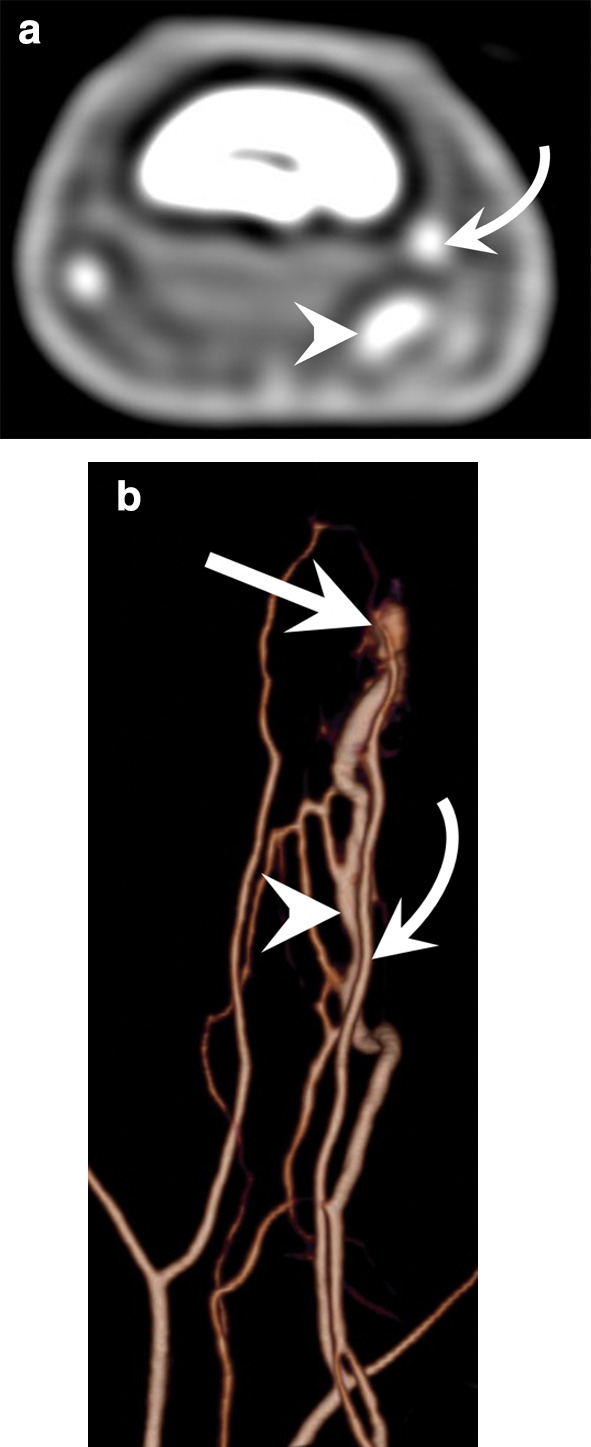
Dynamic CTA of an arteriovenous fistula (straight arrow) fed by the ulnopalmar digital artery (curved arrow) of the fourth finger in a 32-year-old patient. a, b Native slice and VRT image with bone subtraction at the early arterial phase showing the fistula and the early enhancement of an ulnopalmar vein (arrowhead). Dynamic CTA allows capturing the lesion at the best vascular phase
